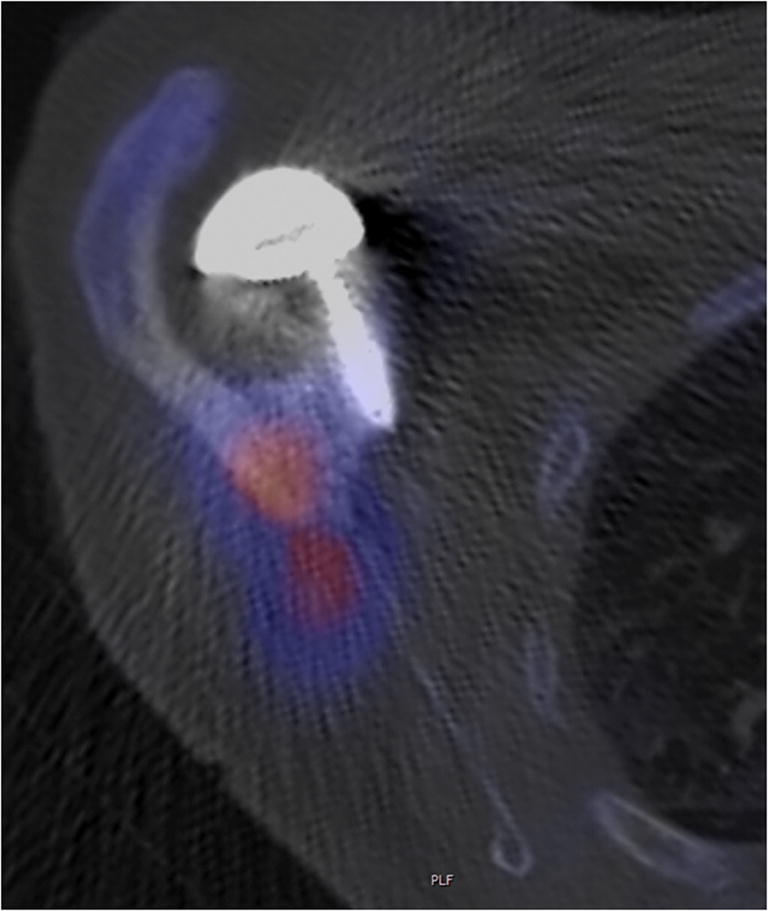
Axial single-photon emission computed tomography (SPECT) image of patient 11 demonstrates a scapular spine fracture on the right side with increased uptake (red area). The superior screw for the fixation of the glenoid base plate was not in the fracture zone
